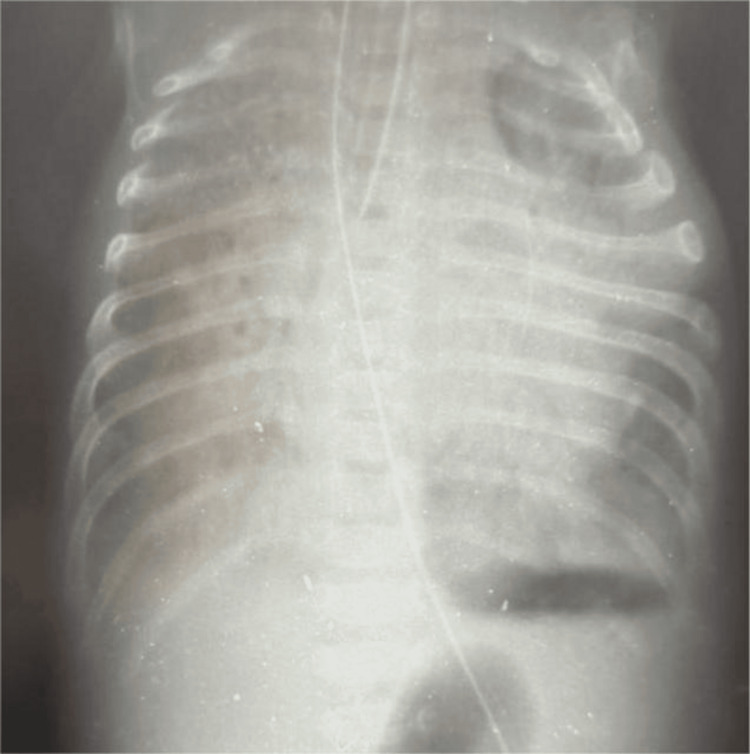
Chest radiograph demonstrating near-complete bilateral lung involvement on the day of death
